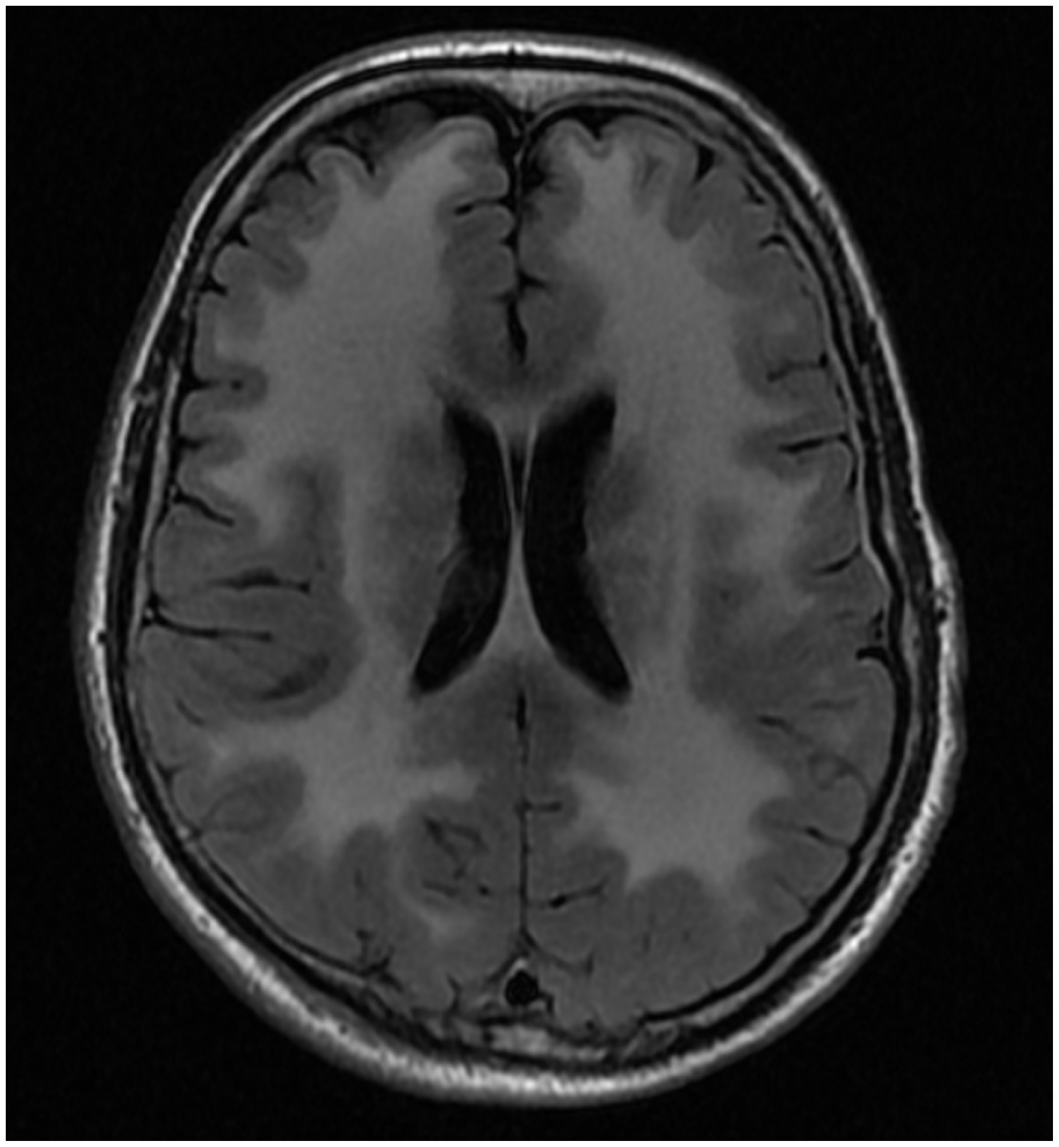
MRI brain of proband. Axial FLAIR^*^ image from MRI brain of proband at 64 years of age showing confluent, bilateral white matter T2# hyper intensity with involvement of the corpus callosum ^*^FLAIR, Fluid-attenuated inversion recovery; #T2, transverse relaxation time.
